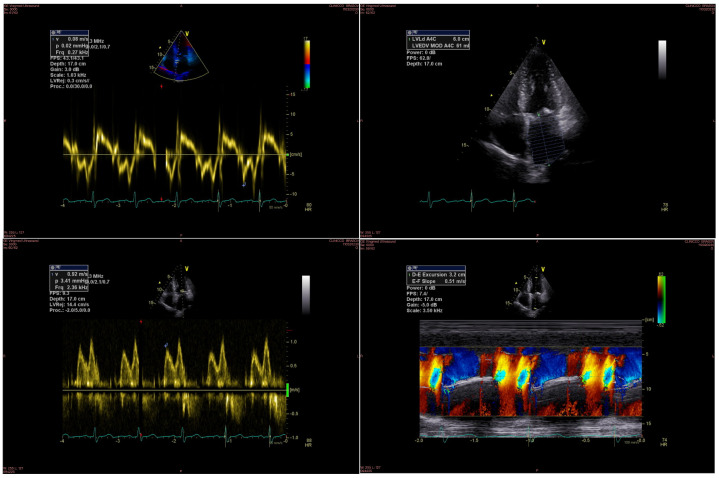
Diastolic function parameters measured by echocardiography: the upper left image shows the Em wave, the upper right image shows the LA volume, the bottom left image shows the E wave, and the bottom right image shows the Vp measurement.
